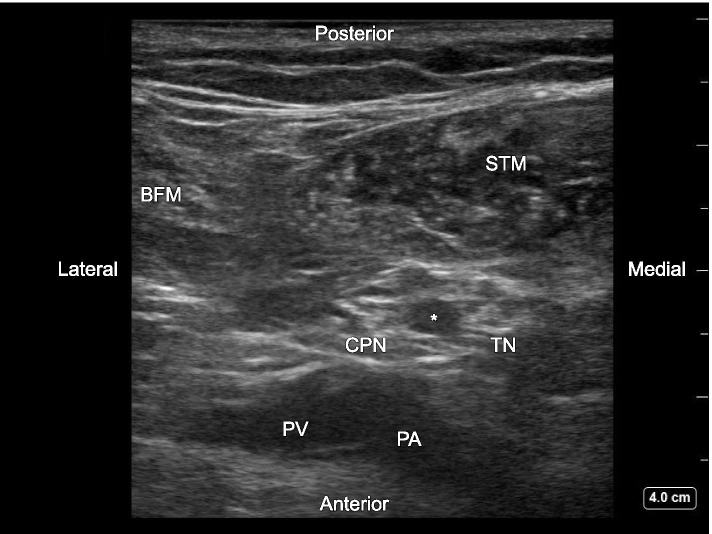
Ultrasound image of an artery within the paraneural sheath between the tibial nerve (TN) and the common peroneal nerve (CPN) at slightly peripheral to the bifurcation of the sciatic nerve. A round hypoechoic pulsating structure (asterisk) was identified between two honeycomb structures (TN and CPN). The diameters of the artery between TN and CPN (asterisk) and the popliteal artery that were measured by the caliper function of the ultrasound machine were 3.2 × 4.2 mm and 5.0 × 6.1 mm, respectively. TN, tibial nerve; CPN, common peroneal nerve; BFM, biceps femoris muscle; STM, semitendinosus muscle; PA, popliteal artery; PV, popliteal vein
